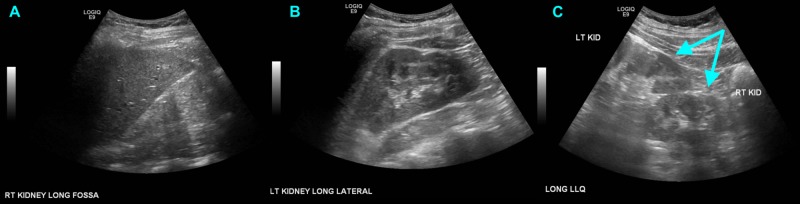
Renal ultrasound performed about one year prior to presentation demonstrating an empty right renal fossa (A), a normal appearing orthotopic left kidney (B), and a fused renal moiety (double arrow) visualized in the left lower quadrant of the abdomen, mistakenly mislabeled as "LT KID" and "RT KID" at the time of scanning (C)
